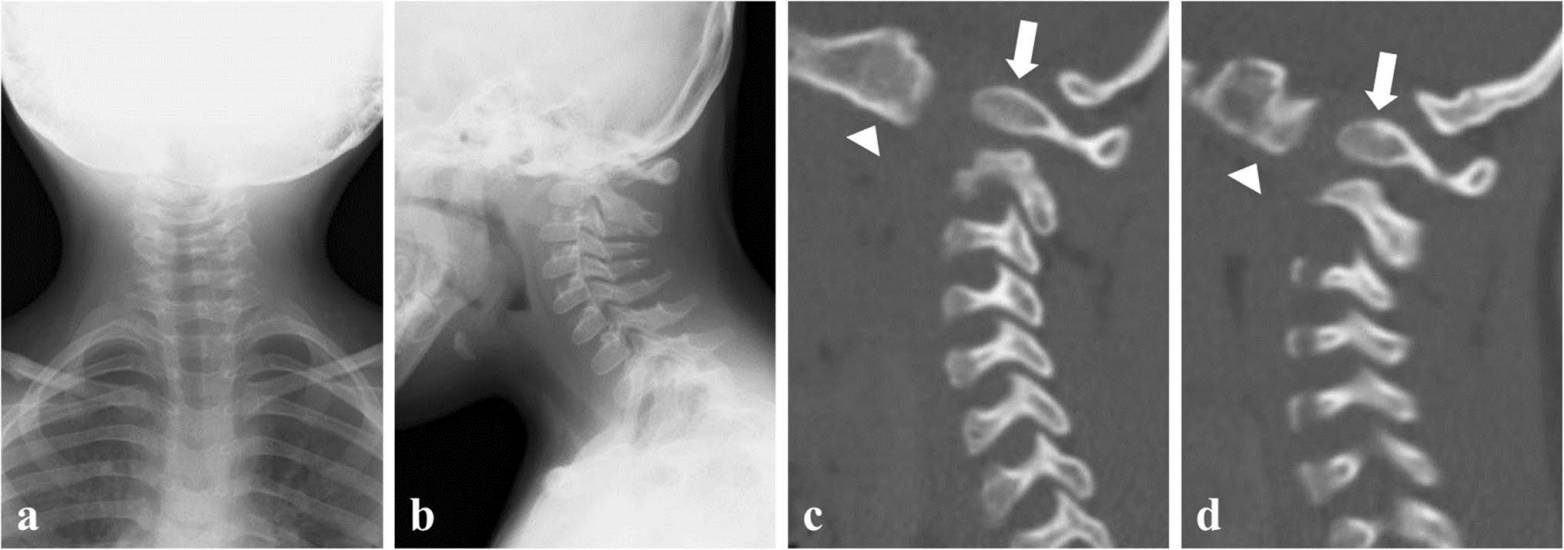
Cervical spine images at 3 years old. a, b Anterior–posterior and lateral plain radiographs of the cervical spine at 3 years old. c, d Reconstructed parasagittal computed tomography of the cervical spine at 3 years old revealing anteriorly displaced right (c) and left (d) occipital condyles (white arrow heads) to the C1 lateral masses (white arrows)
